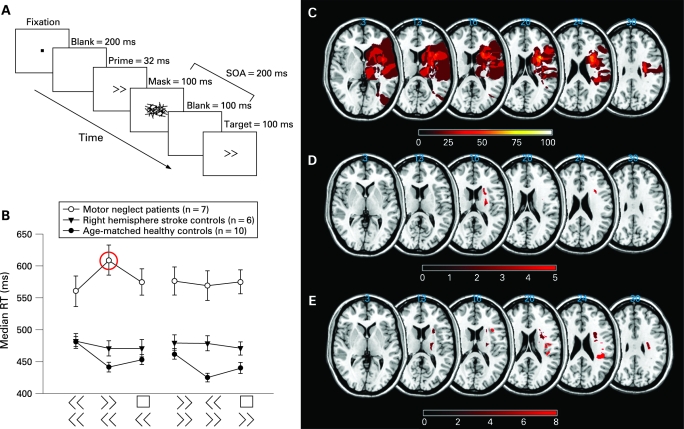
Paradigm, behavioural and lesion data. Subjects performed a masked prime task (A). Arrow stimuli subtended approximately 1.5×1°. Neutral primes comprised the arrows rearranged forming a square that carried no directional information (not shown). Twelve blocks of 24 stimuli contained six different trial types randomised with the constraint that each condition occurred the same number of times per block. Hands were covered during the experiment to prevent visual guidance of movement. Subjects were instructed to fixate the laptop display centrally and eye position was monitored by the experimenter. A practice session (<2 min) took place beforehand. Only patients with motor neglect showed significant reaction time delay when a right prime preceded a movement with the left hand (B, red circle). Hence right hand motor plans significantly intrude on left hand movement, but not vice versa, only in patients with motor neglect. Lesions were plotted using MRICro software (www.mricro.com) from either CT or MR. Lesion subtraction (patients with motor neglect minus those without) shows frontal white matter selectively affected in patients with motor neglect (C). (D) Brunner–Munzel statistic revealed that right putamen and subcortical white matter were significantly associated (z scores >4.47) with abnormal performance in the masked prime task using the left, but not the right, hand. (E) Severity of motor neglect is significantly associated (z >4.49) with damage at several discrete areas within the right hemisphere (including white matter near the putamen, inferior frontal gyrus, rolandic operculum and parietal supramarginal gyrus). RT, reaction time; SOA, stimulus onset asynchrony.
